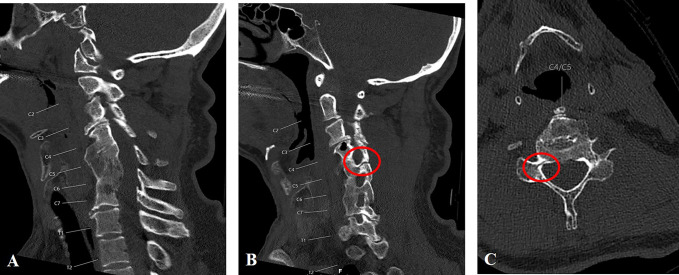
Initial sagittal CT scan demonstrating (A) previous anterior
